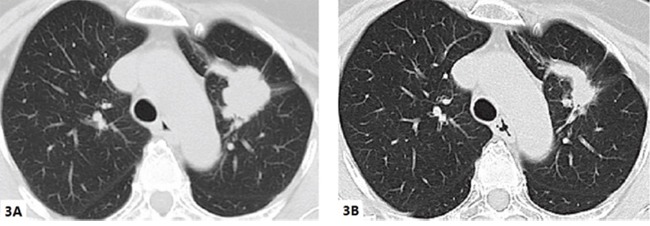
Chest computed tomography of the patient (case 2) before and after icotinib (A) tumor mass in left lung on Aug 26^th^, 2014 (before icotinib): (B) tumor mass in the left lung on Oct 13^th^, 2014 (one and a half month after icotinib).
